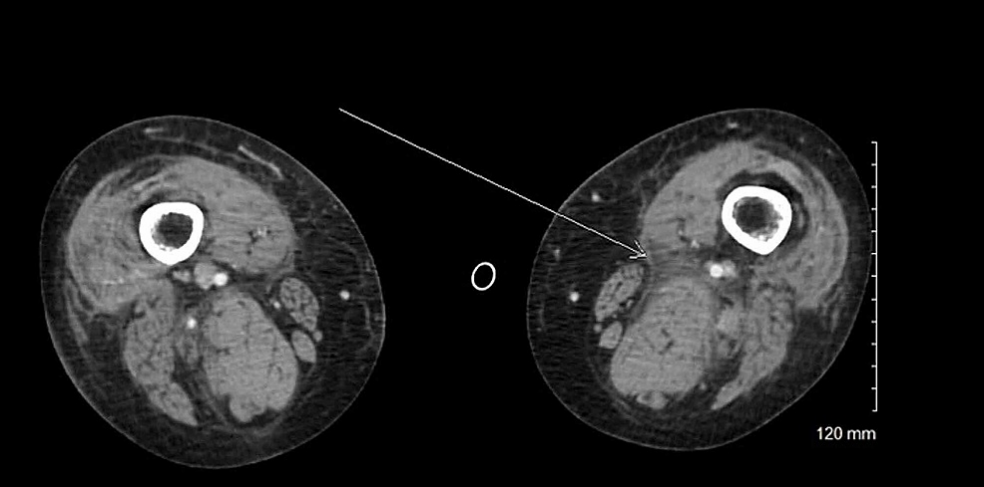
CT scan of bilateral thighs showing subcutaneous tissue edema within the deep muscular compartments.
